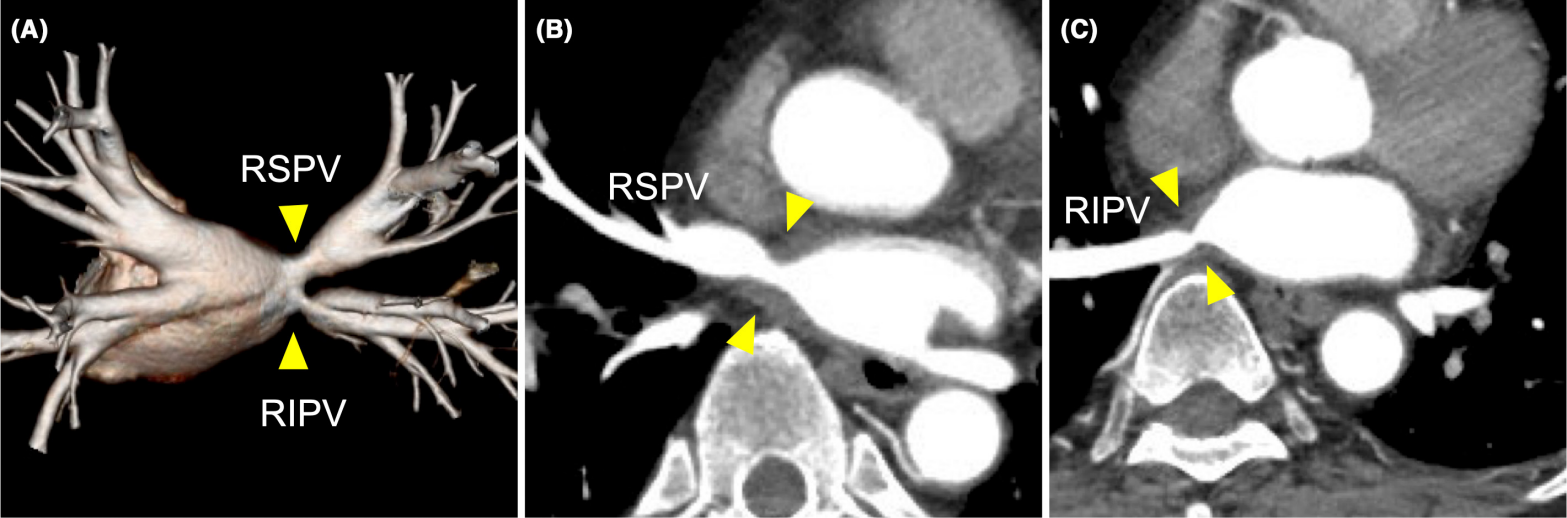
Computed tomography obtained 6 months after ablation. Chronic PV stenosis and thickened wall of ostial PVs were observed.
